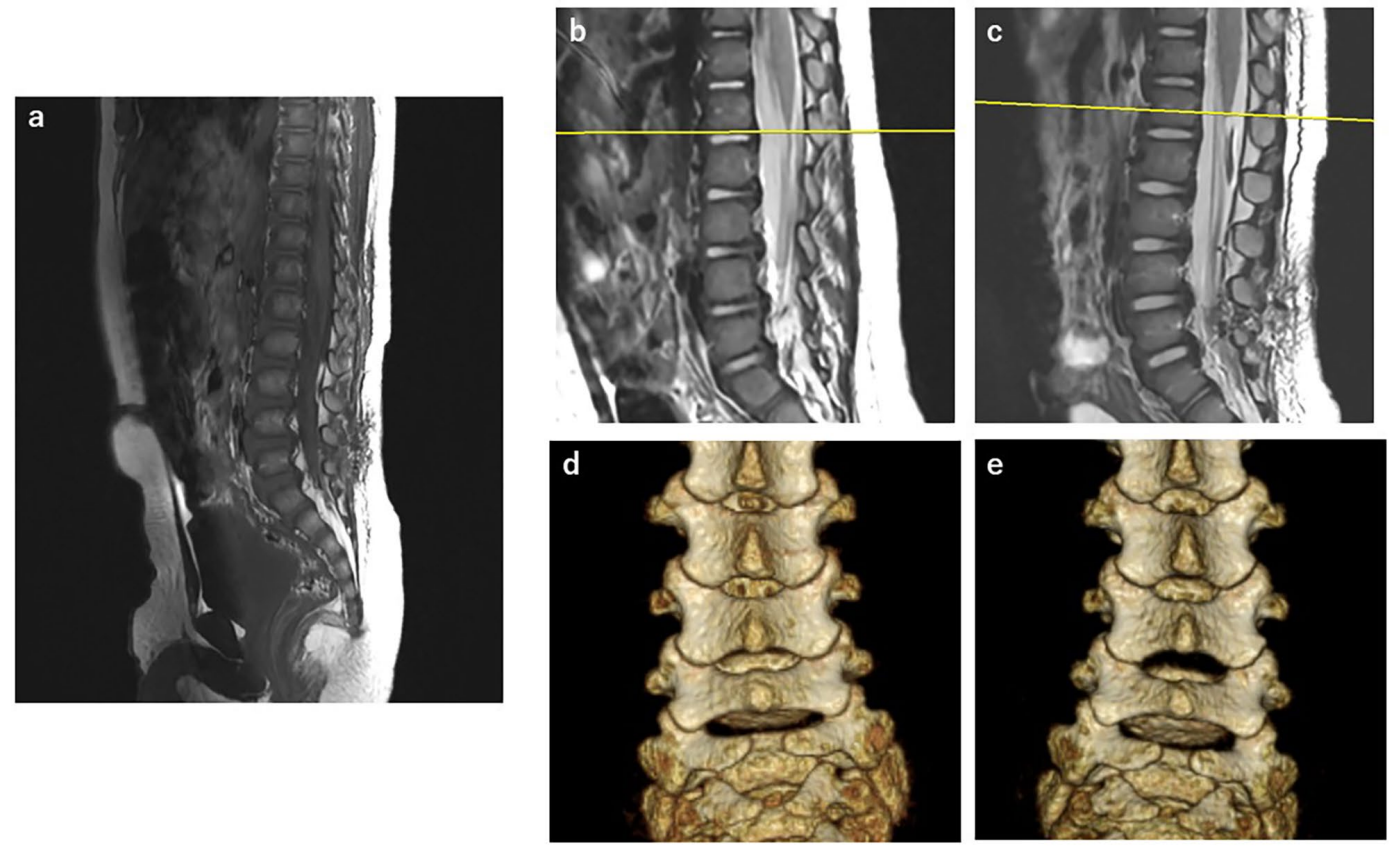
Postoperative Findings. a: Postoperative MRI showed cranial shift of the resected filum terminale. b: Preoperative sagittal T2-weighted image showed the conus level at lower L2. c: Postoperative sagittal T2-weighted image showed the conus level at middle L2. d: Preoperative 3-dimensional computed tomography (3DCT) image of the lumbar spine. e: Postoperative 3DCT image of the lumbar spine
